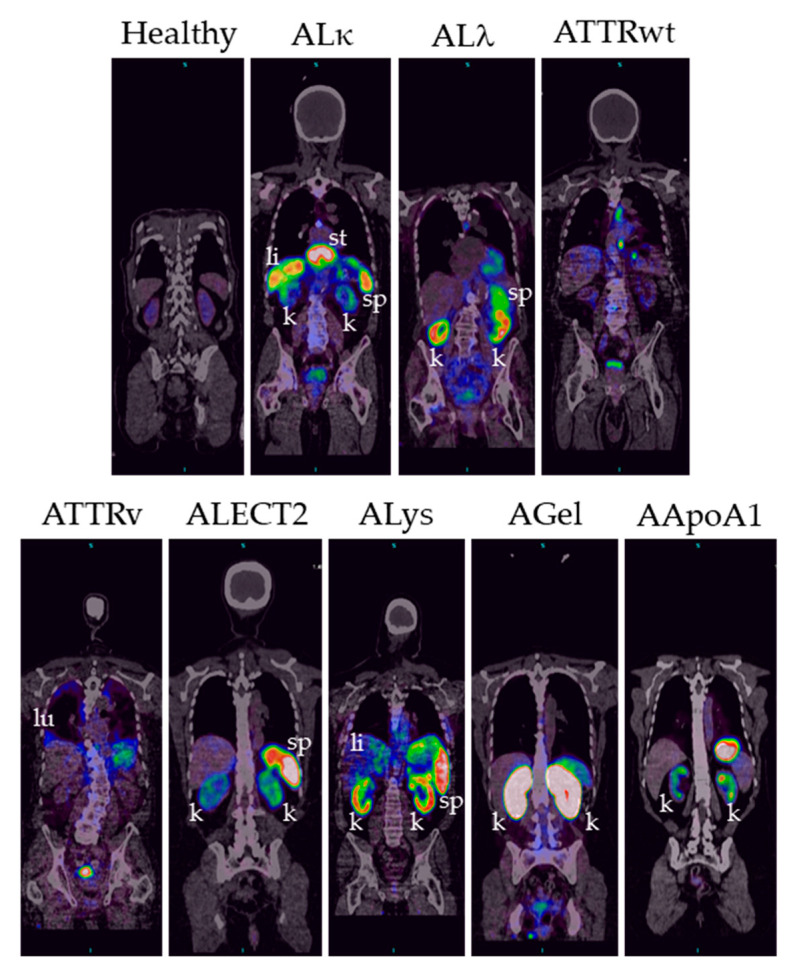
Coronal PET/CT images of a representative healthy subject and patients showing specific and heterogenous uptake of radiotracer in abdominothoracic organs. Images have been scaled as described in Figure 3.
